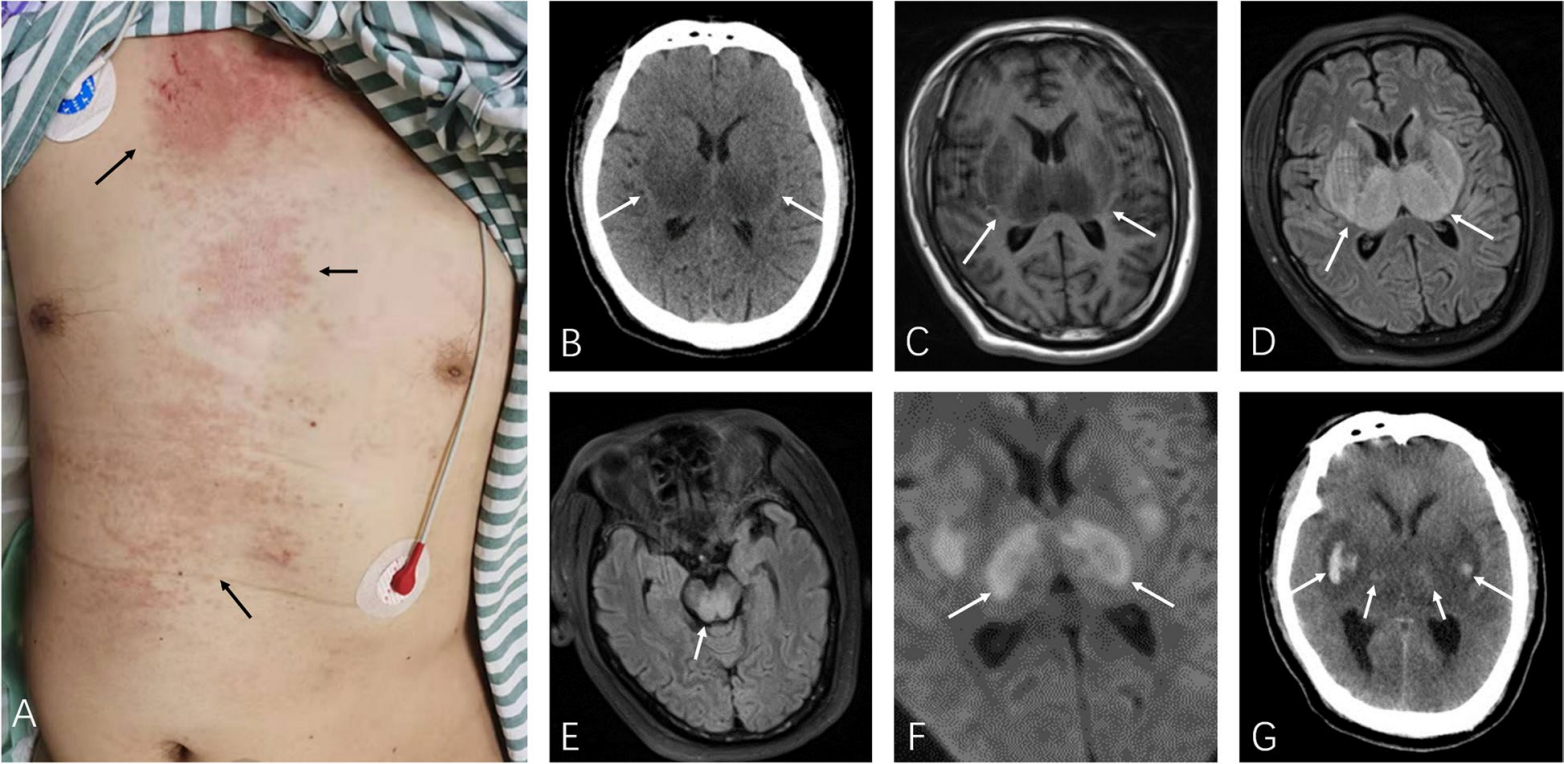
Picture of the patient (A) showing salmon-colored rash in the trunk (black arrows). On hospital day (HD) 1, brain CT (B), T1 weighted (C), and T2-FLAIR (D, E) MRI demonstrating symmetric lesions involving thalami, striatum and brain stem, consistent with acute necrotizing encephalopathy (white arrows). Diffusion weighted images (F) showing typical “concentric/laminar structure”. Brain CT on HD 2 revealing hemorrhage in the lesions (G, white arrows)
